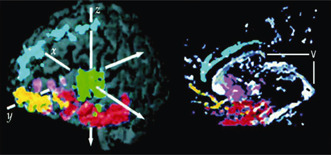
From 37. The 3D [11C](R)‐PK11195 binding potential map projected onto the magnetic resonance image (MRI) of a patient with herpes encephalitis indicates how the inflammation in the temporal lobe and hippocampus (red) is associated with TSPO expression that tracks throughout the limbic system beyond the focal injury. This is likely to be due to Wallerian degeneration along the affected neural tract system (blue = anterior cingulate; green = thalamus and brainstem; purple = insular cortex; yellow = orbitofrontal gyri; the MRI subtraction image in the right panel shows the volume losses, including the enlargement of the ventricles (v) of the destructive tissue pathology).
